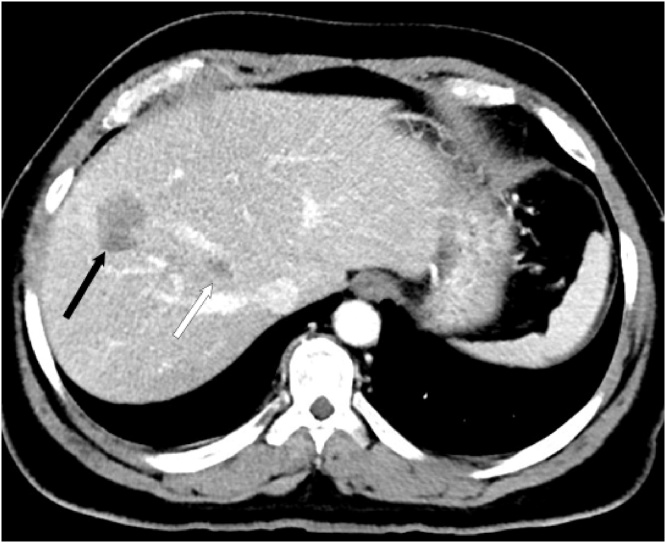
Patient 2: Preoperative contrast-enhanced CT shows new metastasis of the right lobe of the liver (Black arrow). The RFA treated old lesion is marked with a white arrow.
